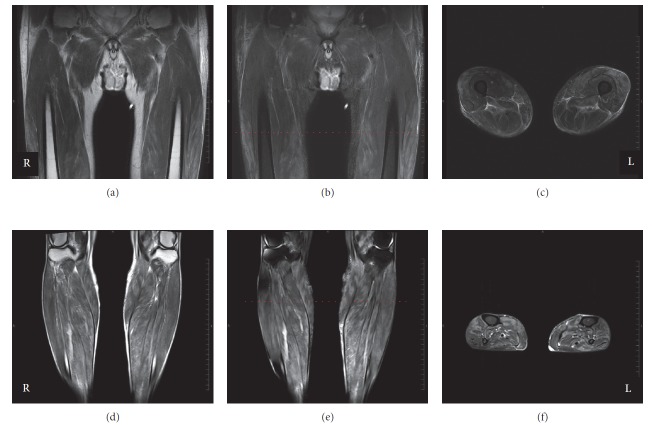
MRI of the lower limbs ((a)–(c) thighs; (d)–(f) legs). T2-weighted ((a) and (d)) and short T1 inversion recovery images (fat suppression method; (b), (c), (e), and (f)). The level of cross-section images ((c) and (f)) is indicated by red dashed lines in the coronal images ((b) and (e)).
